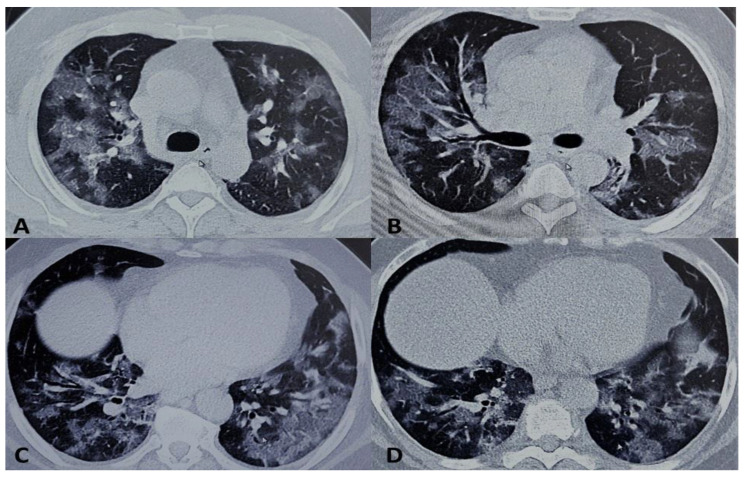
Computerized tomography (CT) scans tocilizumab pre-treatment. (A–D) Thin-slice (1 mm) axial unenhanced CT images demonstrated multiple patchy ground-glass opacity with a peripheral and subpleural distribution. Various reticular opacities are also detected within fields of ground glass (crazy-paving pattern). Carenal and prevascular lymphadenopathy is found.
